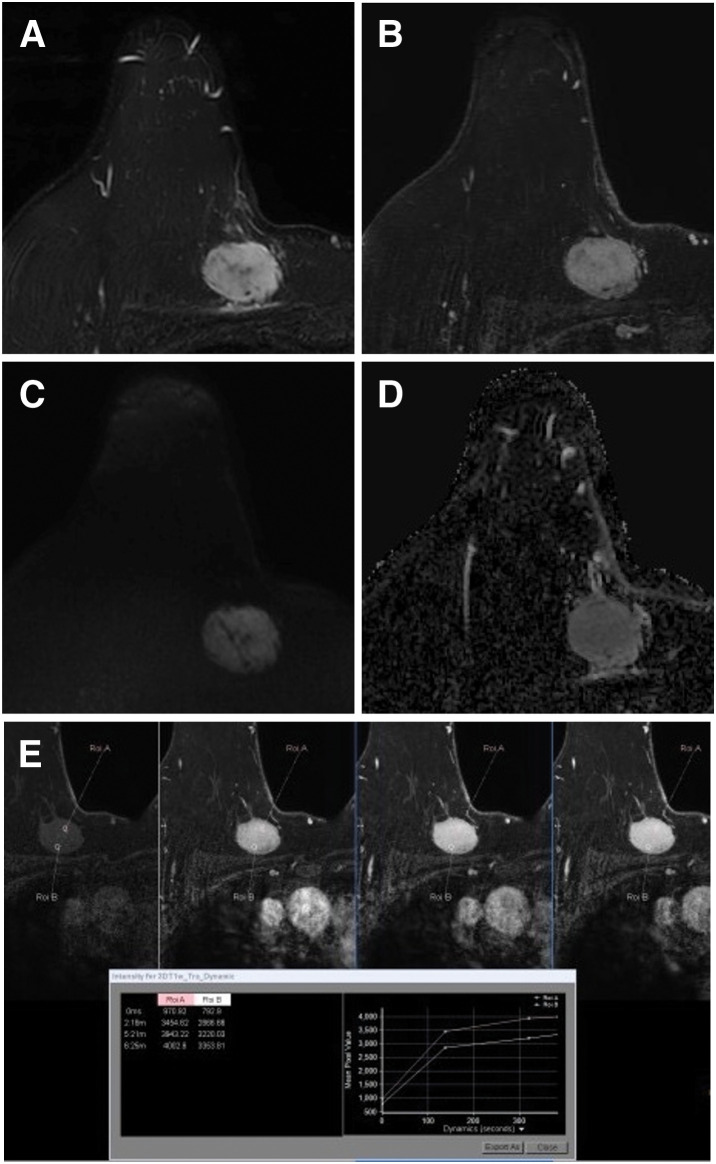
Breast MRI findings. (A) An oval mass with irregular margins showed high signal intensity on fat-suppressed T2-weighted imaging and (B) mild, relatively homogeneous enhancement on early-phase contrast-enhanced T1-weighted imaging. (C) Diffusion-weighted imaging and (D) the apparent diffusion coefficient map show no definite diffusion restriction. (E) The time–signal intensity curve showed a fast–persistent enhancement pattern.
